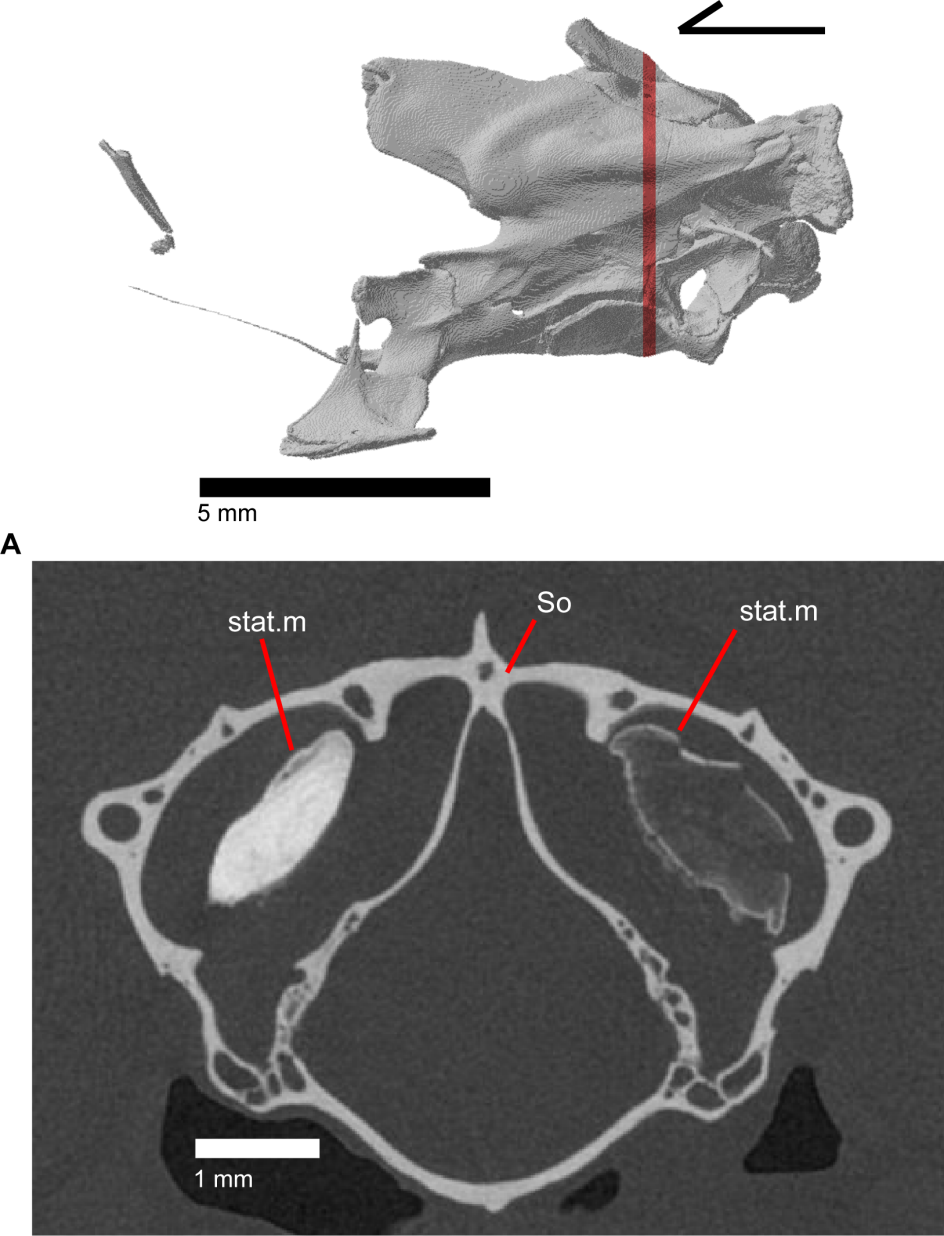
CT coronal slice of *Elgaria panamintina* MVZ 75918.
